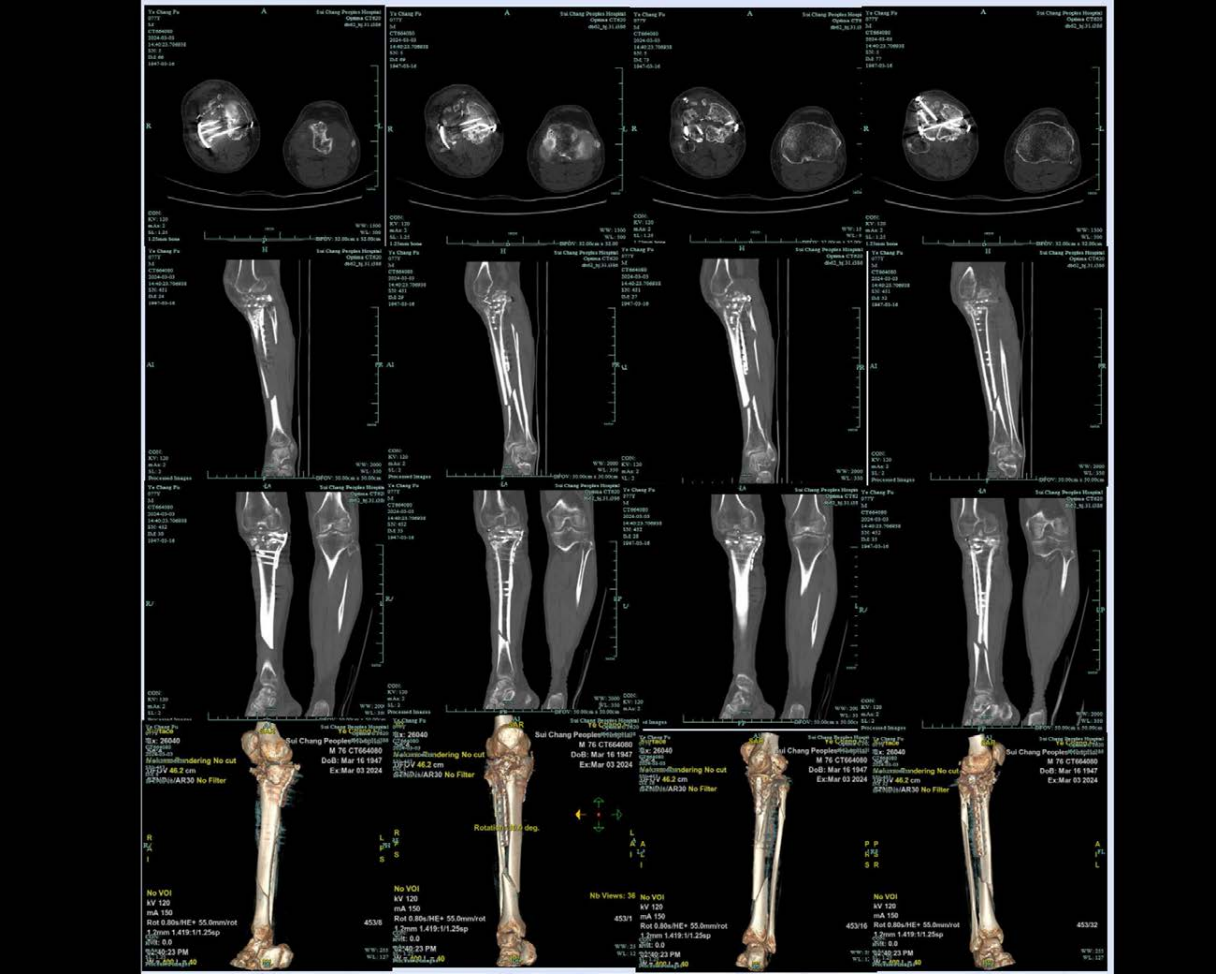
Computerized tomography showed fracture of the tibia and fibula with persistent nonunion of the TPF (Fig. 8; date of examination: March 3, 2024). Axial (A; B; C; D), agittal (E; F; G; H), coronal (I; J; K; L), and 3-dimensional (M; N; O; P) CT.
